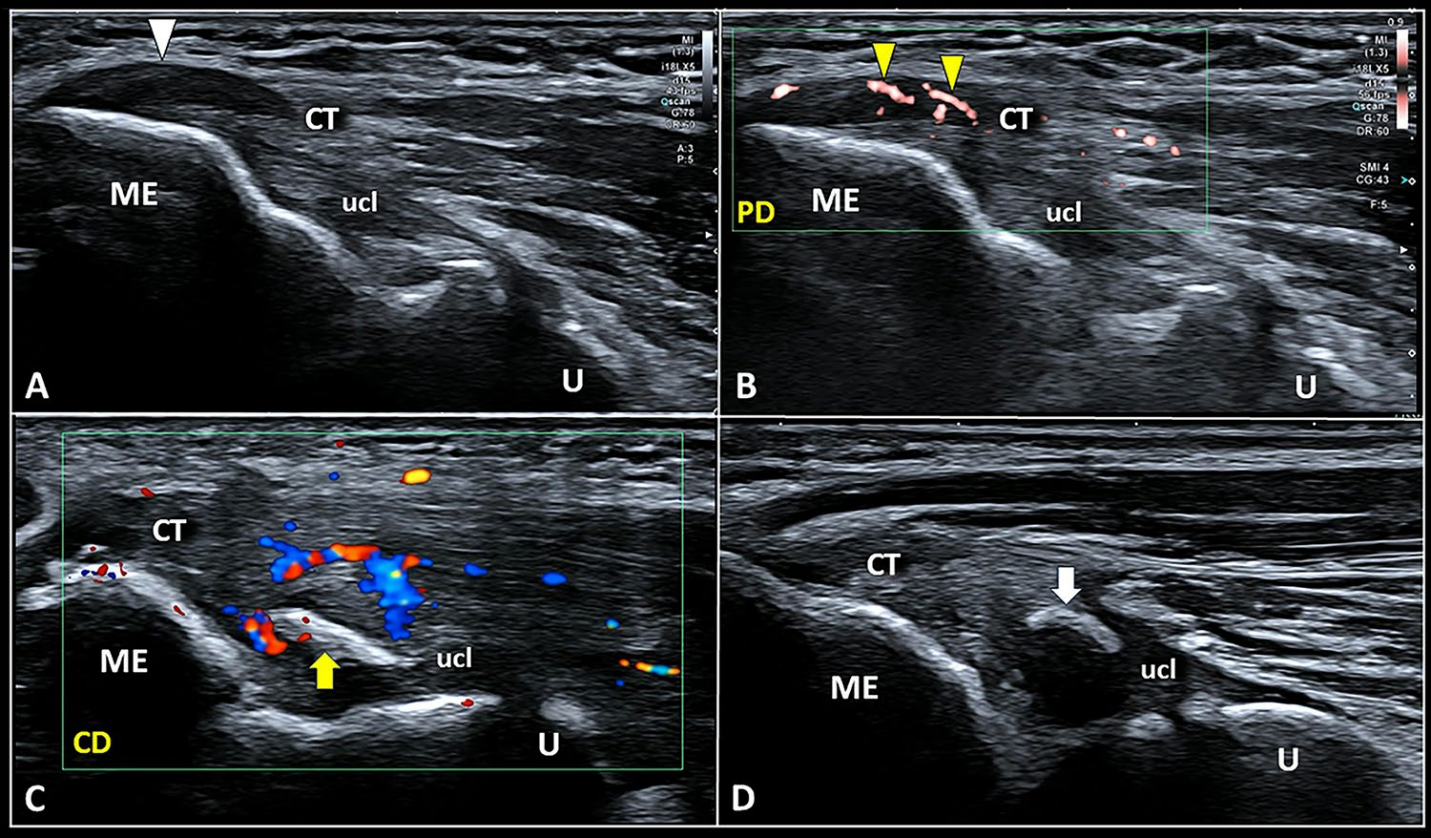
Hypoechoic thickening *(white arrowhead)* of the conjoint tendon *(CT)* in B-Mode (A) with neo-vessels *(yellow arrowheads)* penetrating the degenerated tendon tissue in power Doppler *(PD)* (B) are common sonographic findings in padel players with medial elbow pain. More rarely, a small bony fragment *(yellow arrow)* embedded in the ulnar collateral ligament *(ucl)* and surrounded by an intense hypervascularization (C) can be observed close to the painful medial epicondyle *(ME)*. Of note, a small ossicle *(white arrow)* within the collagen fibers of the ulnar collateral ligament *(ucl)*, commonly rounded in shape and not encircled by neo-vessels, can be identified also in asymptomatic volunteers (D). *U* ulna, *CD* color Doppler
